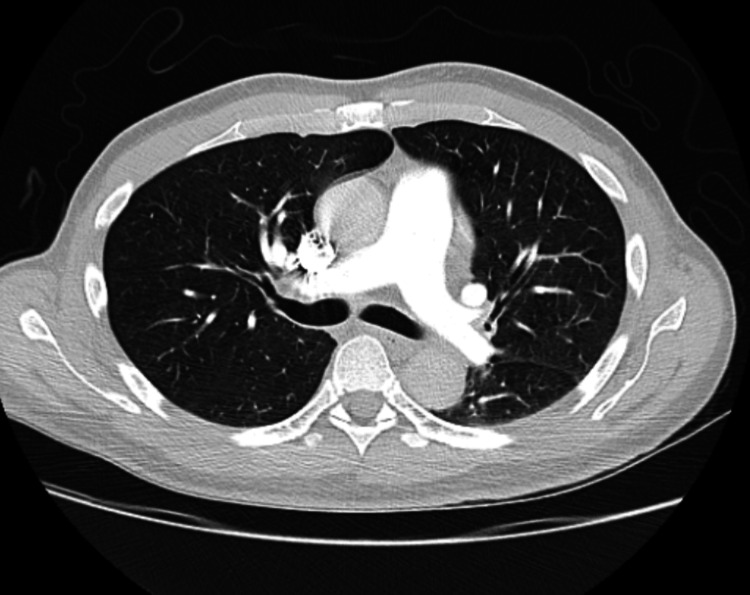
Axial computed tomography of the chest after completion of treatment with re-expansion of the left upper lobe
